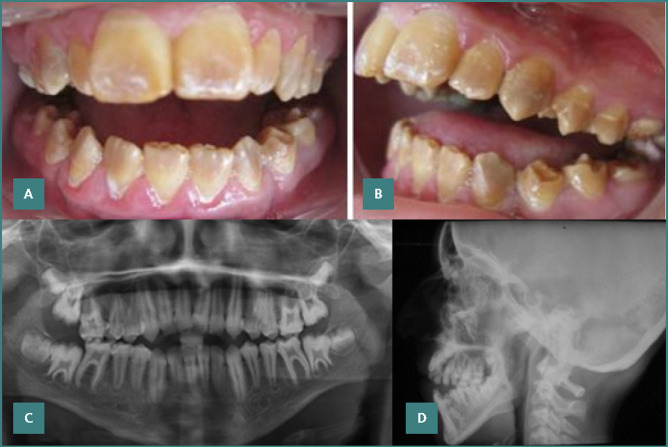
Initial Clinical presentation and radiographic assessment. A, Intraoral front view showing the yellow color of the teeth and rough enamel surfaces. B, Intraoral right lateral view revealing severe anterior open bite. C, Orhopantomographic radiograph. D, Lateral cephalogram radiograph.
